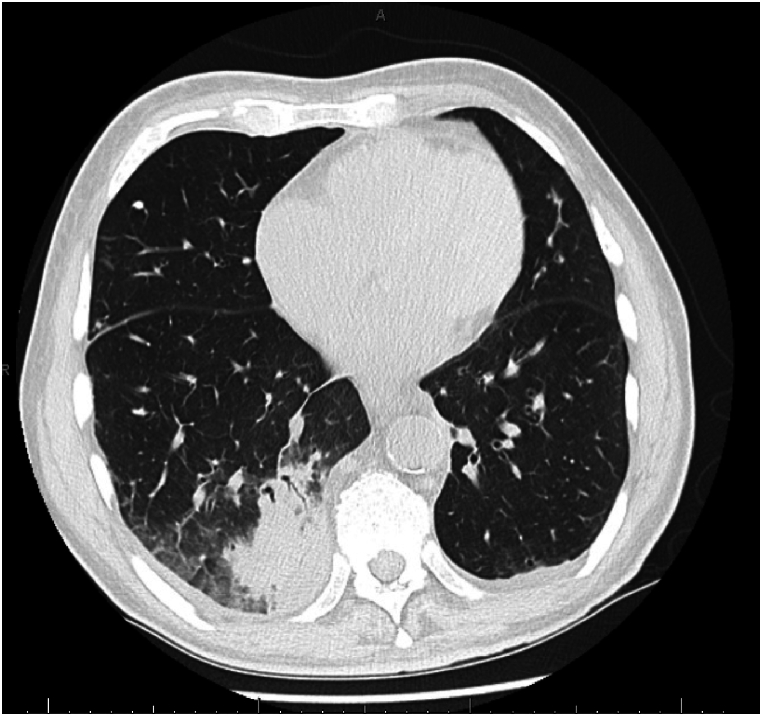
Representative image from CT chest without IV contrast showing right lower lobe consolidation with air bronchograms and surrounding ground glass opacity.
